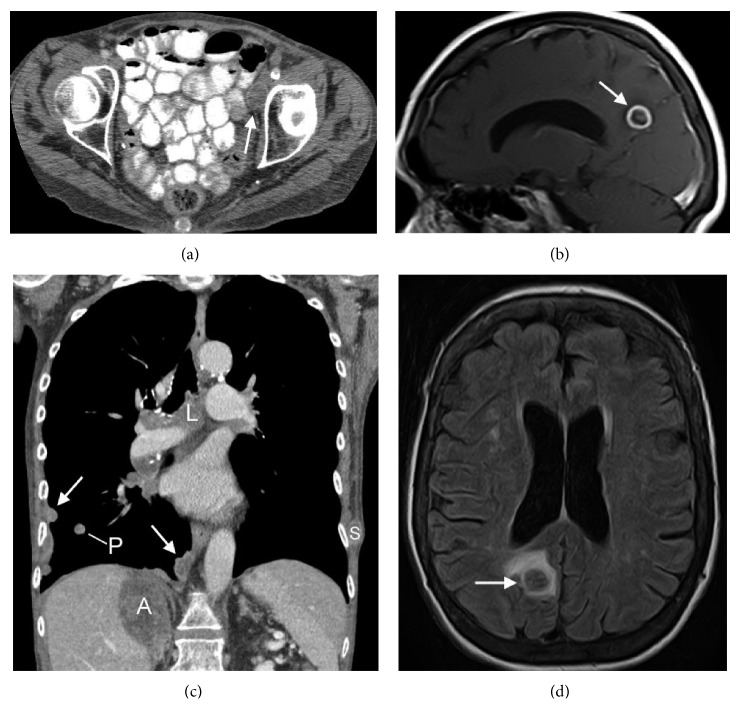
Progression of disease 18 months after starting capecitabine treatment. Axial postcontrast CT of the pelvis (a) shows increased size of a centrally necrotic left external chain lymph node, measuring 2.2 × 1.7 cm (white arrow). Postcontrast sagittal T1 MRI (b) and noncontrast coronal FLAIR MRI (d) of the brain show a new rim enhancing 1.2 cm metastasis in the right posterior parietal lobe with surrounding edema (white arrows). Coronal postcontrast CT of the chest and abdomen (c) shows new right pleural metastasis (arrows), mediastinal and hilar lymph node (L), and left lateral chest wall soft tissue (S) metastases, as well as an enlarging right lower lobe pulmonary metastasis (P) and right adrenal metastasis superimposed on an existing adrenal adenoma (A).
